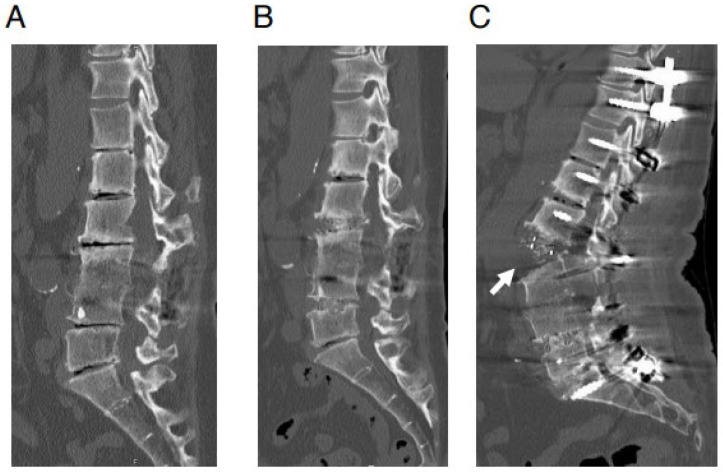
ALL rupture during posterior correction procedure: representative case. A 64-year-old woman with adult spinal deformity. In this case, postero-lateral fusion had been performed at L3/4 level previously at another hospital. Preoperative and postoperative reconstructed sagittal views of CT images. (A) Preoperative CT image. (B) CT image after OLIF (L2/3·L4/5). (C) CT image after posterior correction procedure. In this case, surgery was performed when only the less lordotic (6°) OLIF cages were available. Anterior longitudinal ligament rupture (white arrow) is observed after the posterior correction procedure.
